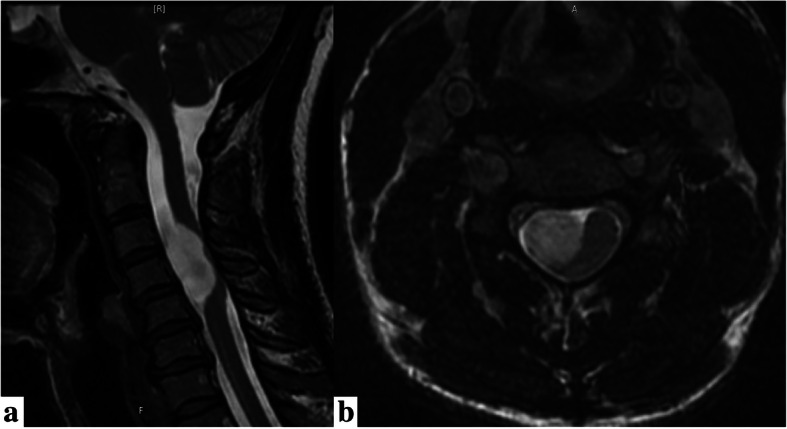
a Sagittal and b axial preoperative T2 MRI
